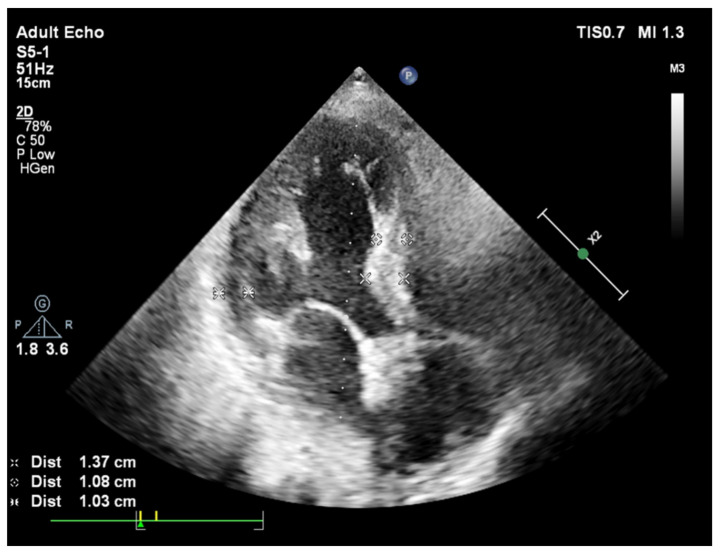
A slight curve of septal base from apical 4 chamber view during end-diastole in a patient with systemic hypertension and basal septal hypertrophy.
